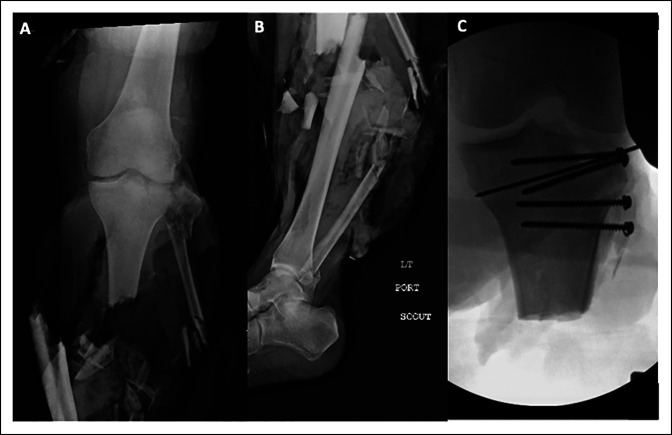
A and B, Original injury radiographs depicting Gustilo-Anderson grade IIIC open tibia and fibula diaphyseal fractures with severe soft-tissue injury and partial limb amputation (OTA classification 42-C3). There is a proximal fibular fracture with proximal tibiofibular joint dissociation and a simple split fracture of the lateral tibial plateau articular surface. C, Intraoperative fluoroscopic radiographs demonstrating revision amputation of the injured extremity with open reduction and internal screw fixation of the lateral tibial plateau split fracture in addition to open reduction and internal fixation of the proximal tibiofibular joint with 3.5 mm cortical screws.
